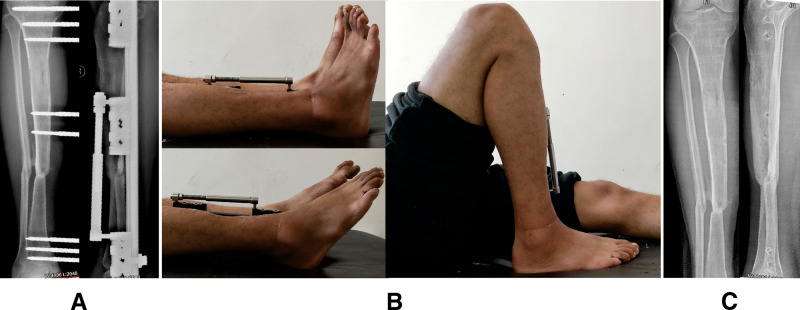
Images of the same patient shown in Figure 2. (A) Radiographs reveal the complete consolidation and docking site union. (B) General appearance before frame removal, showing the satisfactory range of motion of knee and ankle joint results. (C) Radiographs 6 months later after removing the external fixator.
